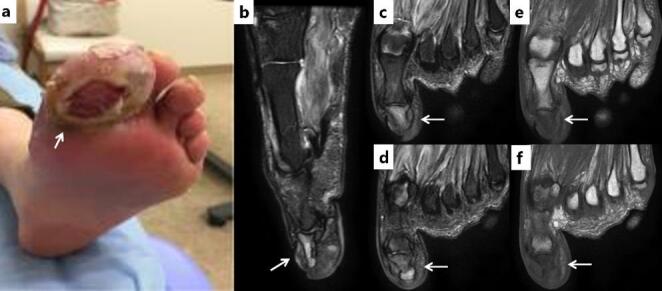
Osteomyelitis. a Gross appearance, b–f MRI: b STIR (sagittal image), c–d STIR (coronal image), e–f T1WI (coronal image). A woman in her 50s with type 2 DM presented with cellulitis of the left lower extremity and ulceration of the great toe (a). The bone marrow of the distal phalanx shows high signal intensity on STIR images and low signal intensity on T1WI images (b–f; arrow). Osteomyelitis caused by an ulcer was suspected, and the patient’s symptoms improved with antibiotic therapy. Abbreviations: MRI, magnetic resonance imaging; STIR, short-tau inversion recovery
